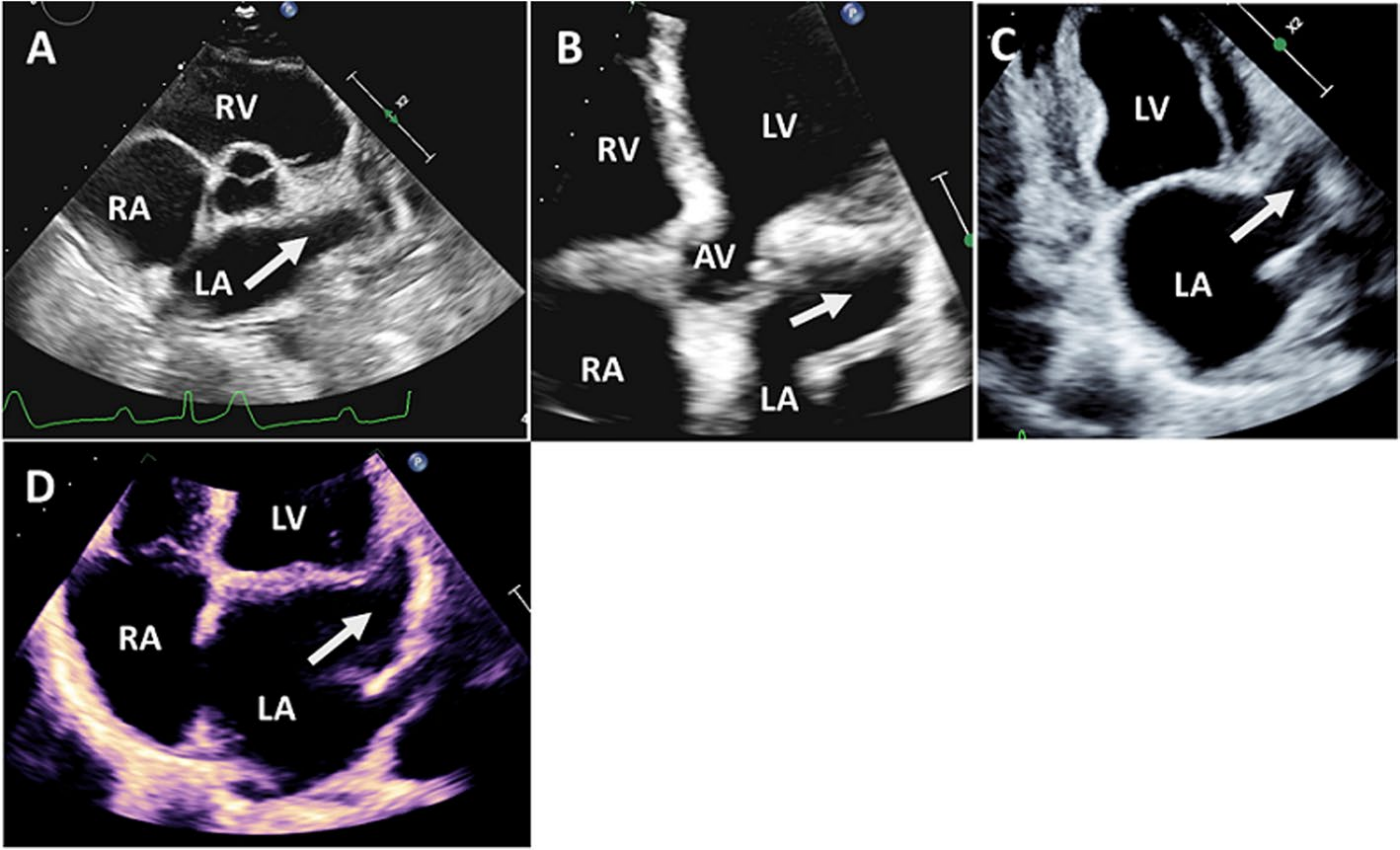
Visualization of the left atrial appendage (white arrow) using two-dimensional transthoracic echocardiography. A From the parasternal short-axis view at the aortic valve level. (B) Apical five-chamber view. C Apical two-chamber view. D Apical four-chamber view
